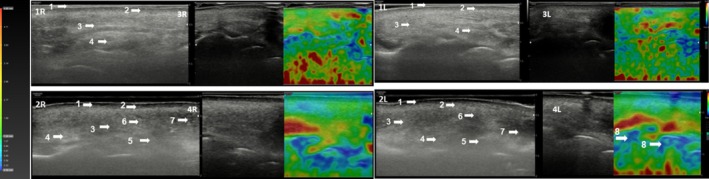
Ultrasound images of the right (R) and left (L) nasolabial folds at baseline (1R & 1L) and at 6 months post‐initial treatment (2R & 2L). Qualitative elastography images of the same region are shown at baseline (3R & 3L) and at 6 months (4R & 4L) in the patient presented in Figure 6. At baseline (1R & 1L), ultrasound revealed a heterogeneous pattern of the nasolabial fold. By month 6 (2R & 2L), there was a notable increase in tissue density at NLF points 1–2–3, evidenced by the following changes: Increased hyperechogenicity is evidenced by the presence of hyperechoic (white) signals within a heterogeneous pattern (5, 6) indicating enhanced tissue density and elevated elastic modulus associated with collagen production (white and light areas). A reduction in the Sub‐Epidermal Low Echogenic Band (SLEB), along with increased echogenicity, further reflects higher tissue density (5, 6), although some residual heterogeneity remains (7). Evidence of neocollagenesis at the level of the reticular dermis, particularly at its interface with the subcutaneous tissue (5, 6). Areas of increased viscoelasticity, corresponding to a higher elastic modulus, were visualized in blue on qualitative elastography (8). 1. Epidermis; 2. Dermis; 3. Subcutaneous Cellular Tissue; 4. Fibrillar Collagen areas; 5. Neocollagen; 6. Hyperechoic Pattern; 7. Anec/Hypoechoic areas; 8. Areas of increased viscoelasticity.
